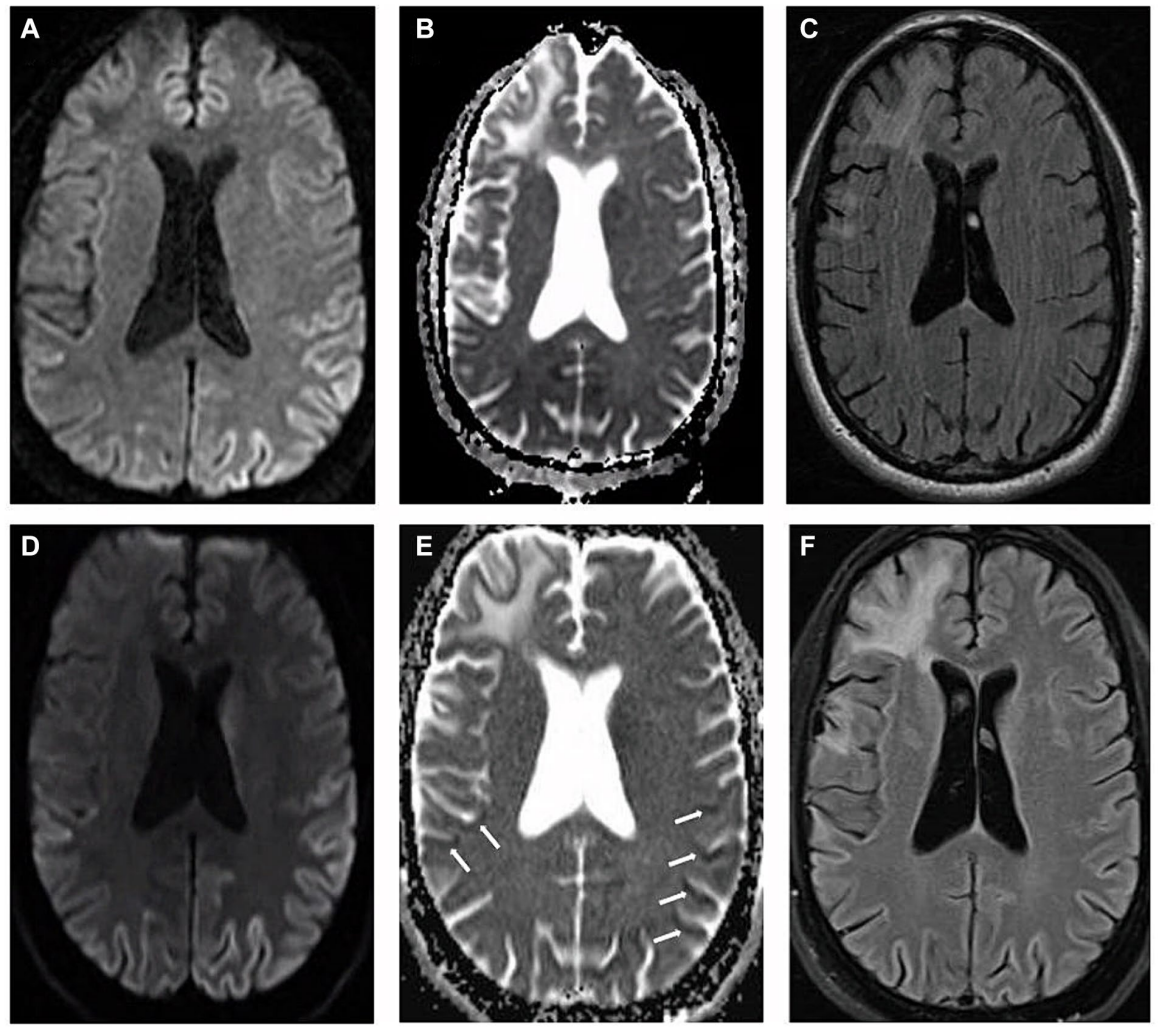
Magnetic resonance imaging: (A–C) represent first MRI scan in March, 2022 and (D–F) demonstrate second MRI scan acquired two months afterwards. (A,D) Axial diffusion weighted imaging (B,E) axial apparent diffusion coefficient imaging (C,F) axial T2 fluid attenuated inversion recovery imaging. White arrows indicate the locations of apparent diffusion coefficient correlate.
